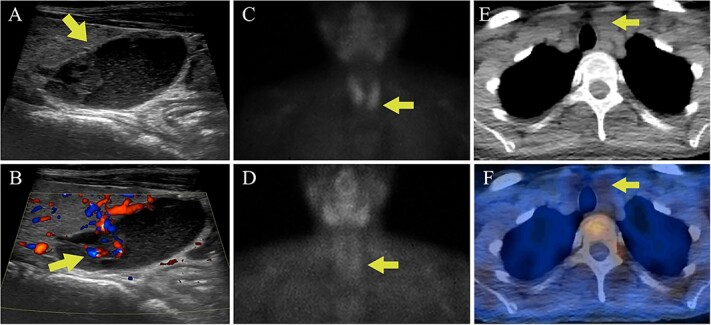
Case 2 parathyroid localization studies. (A) Thyroid US revealed a left inferior thyroid lobe lesion measuring 1.5 cm deep × 1.6 cm wide × 2.0 cm long (arrow, sagittal view). The lesion had a complex cystic and solid appearance with layering internal debris. (B) The solid component of the lesion demonstrated vascularity (arrow, sagittal view with Doppler). (C) Sestamibi scintigraphy showed physiologic radiotracer uptake of the thyroid gland on the immediate images (arrow). (D) One hour delayed sestamibi images showed radiotracer washout without areas of focal sestamibi retention (arrow). (E) The lesion observed on thyroid US correlated with a hypoattenuating lesion posterior to the left thyroid lobe on the CT scan that was done for (arrow). (F) The hypoattenuating lesion did not have increased radiotracer uptake on the fused SPECT/CT image (arrow).
